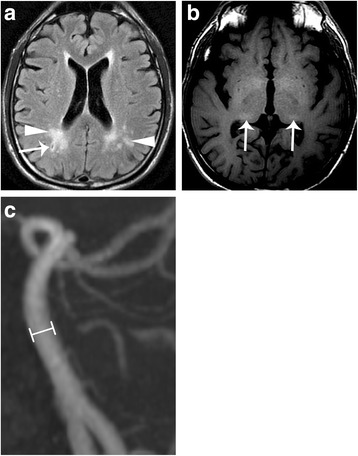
A middle-aged man with classical-type Fabry disease who suffered from chronic renal disease. a Brain magnetic resonance axial T2 fluid-attention inversion recovery reveals an old lacunar infarct at the right centrum semiovale (arrow) and deep white matter hyperintensities (arrowheads). b Axial T1-weighted image reveals high signal changes at the bilateral posterior thalamus (arrows), the pulvinar sign. c The diameter at the middle segment of the basilar artery (lines) was measured as 4.0 mm on a three-dimensional time-of-flight magnetic resonance angiogram
